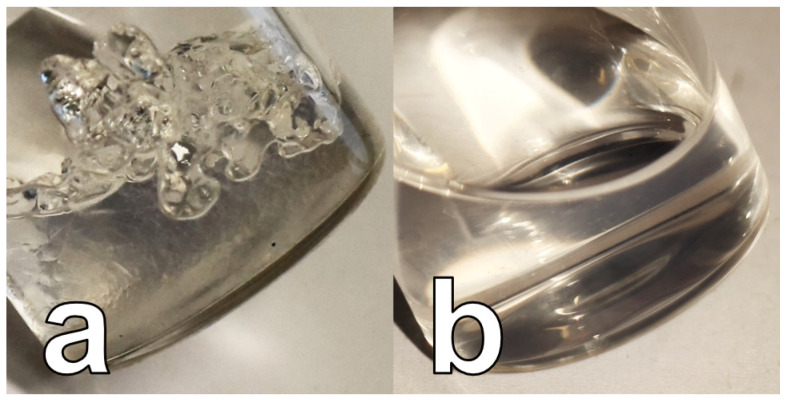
Photo of (a) the coarse-grained alcogel, and (b) alcogel dispersion after ultrasonic treatment.
